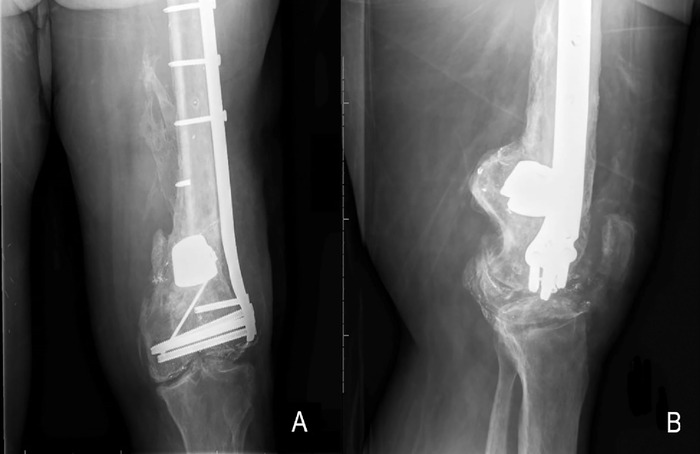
(A) Anteroposterior and (B) lateral radiographic films at final evaluation, more than 7 years after the patient's initial injury and after superficial femoral artery endarterectomy, show osseous healing of the distal femur with no signs of hardware loosening.
